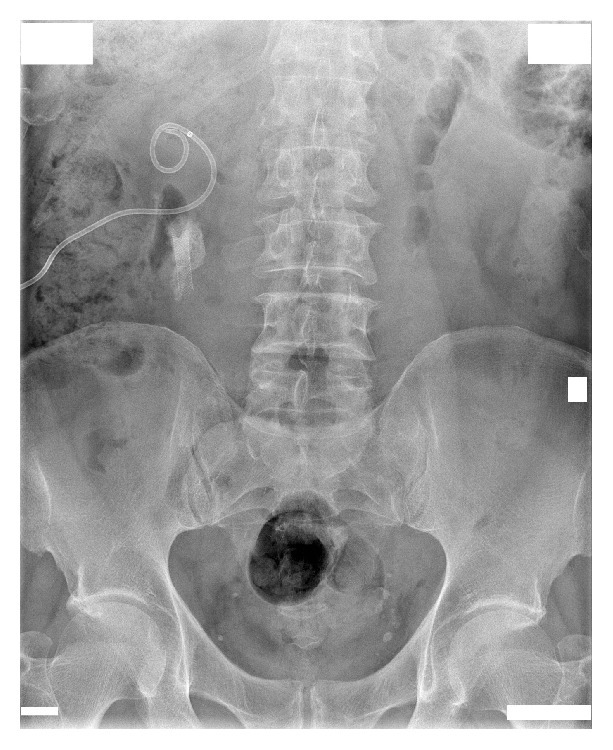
Plain X-ray image of the urinary tract after eight extracorporeal shock wave lithotripsy sessions shows partial disintegration of the stone in the right renal pelvis.
